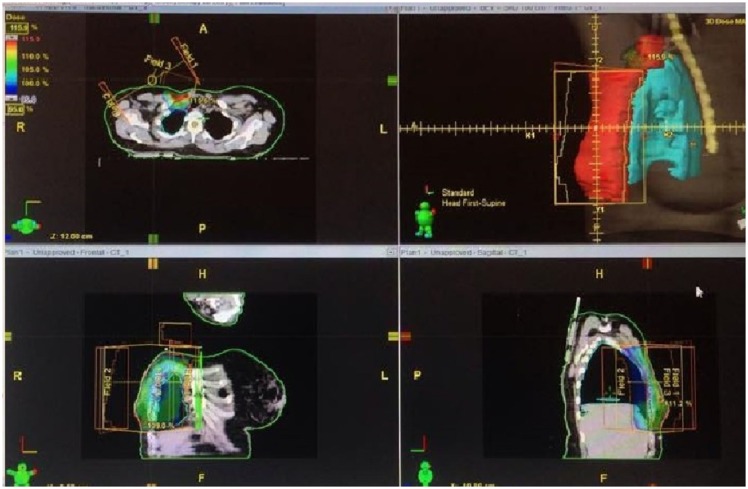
CT planning for a patient in our study showing the field arrangements, doses.
